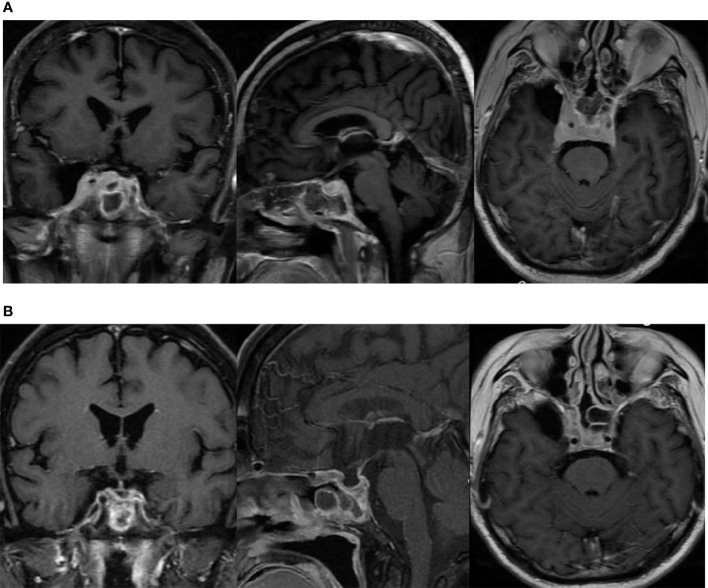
The lesion in the sellar region involving bilateral cavernous sinus as shown in MRI. (A) before chemotherapy; (B) after two courses of chemotherapy (R^2^-MTX).
